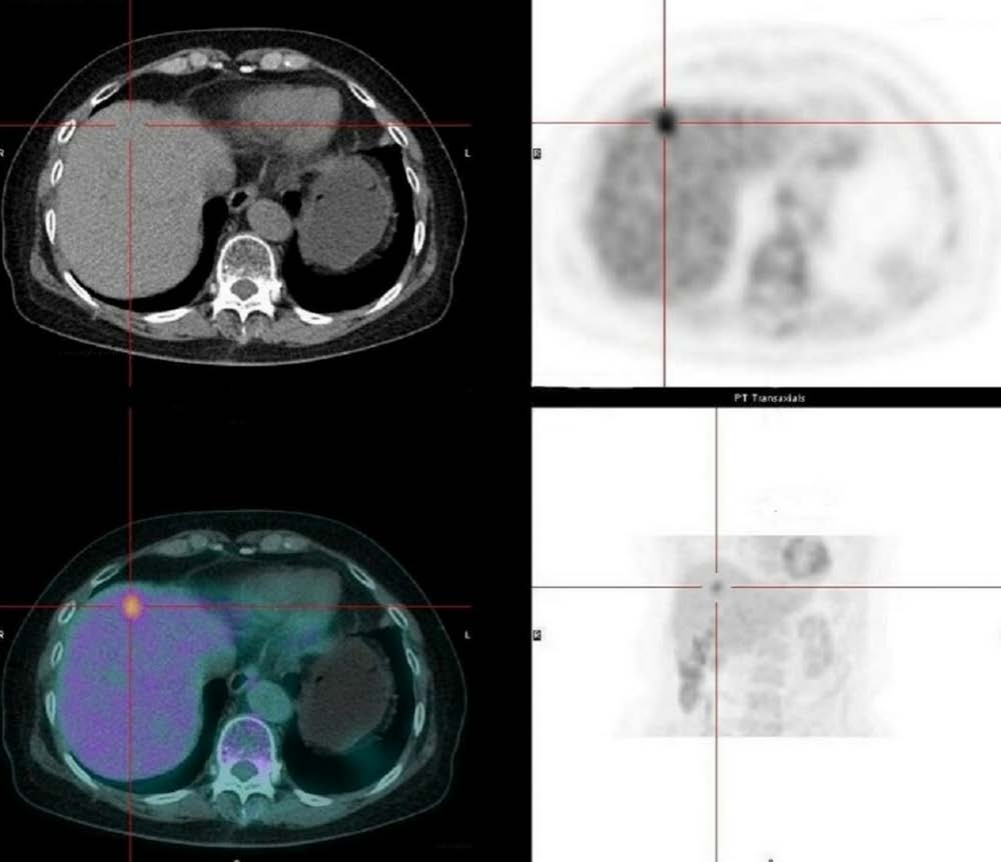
A slightly low-density lesion with increased FDG uptake was seen at the upper margin of the left medial lobe. FDG = fluorodeoxyglucose.
